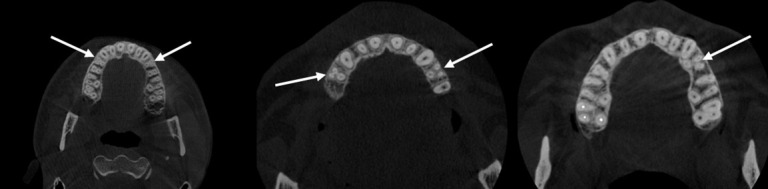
CBCT (Cone-Beam Computed Tomography) in the axial plane revealed three-rooted maxillary first premolars (arrows indicate the examined teeth)
